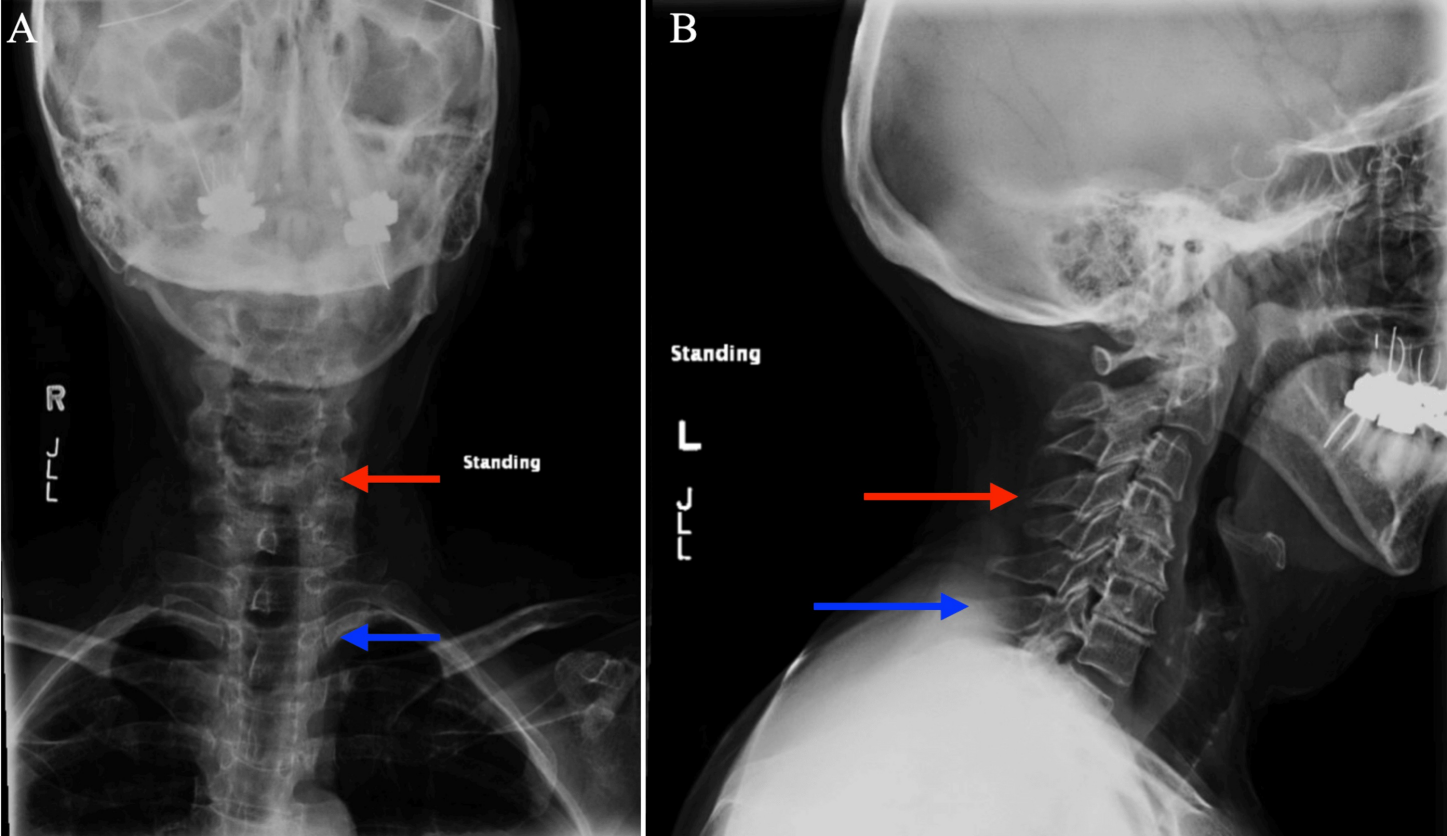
Coronal (A) and sagittal (B) x-ray images of the cervical spine demonstrating degenerative changes from C4 through C7. The red arrows mark the C4 vertebrae and the blue arrows mark the C7 vertebrae.
